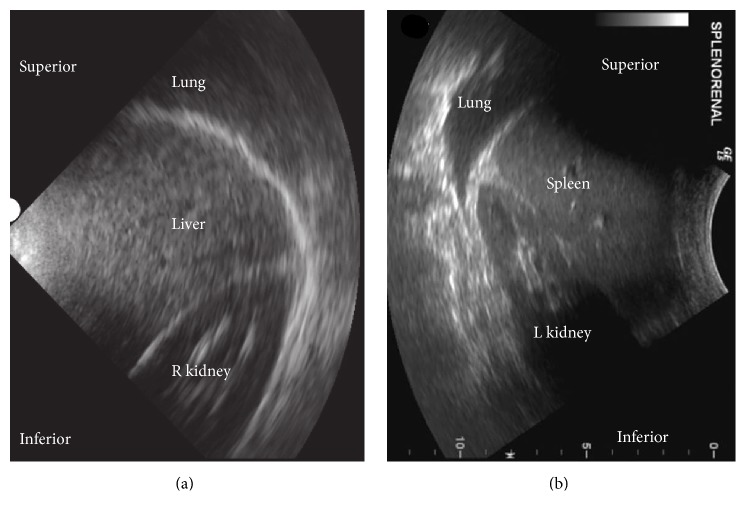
Renal assessment: ultrasound landmarks for kidneys. (a) RUQ hepatorenal view: landmarks for locating the kidney in the lateral right upper quadrant using a phased-array probe. The echogenic line separating the lung from the liver is the diaphragm (see Figure 4, probe position B). Adapted from Perera et al. with the authors' permission [27]. (b) LUQ splenorenal view: landmarks for locating the kidney in the lateral left upper quadrant using a curvilinear probe. The echogenic line separating the lung from the spleen is the diaphragm (see Figure 4, probe position C). Adapted from Montoya et al. with the authors' permission [1].
